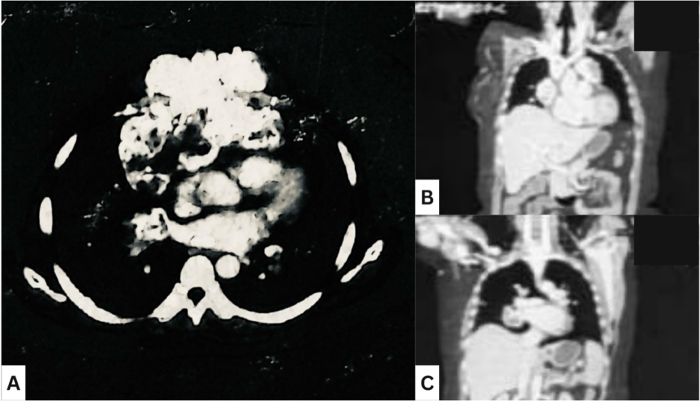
CT scan post-chemotherapy. (A) Transverse cut. (B, C) Coronal cut post-chemotherapy.
